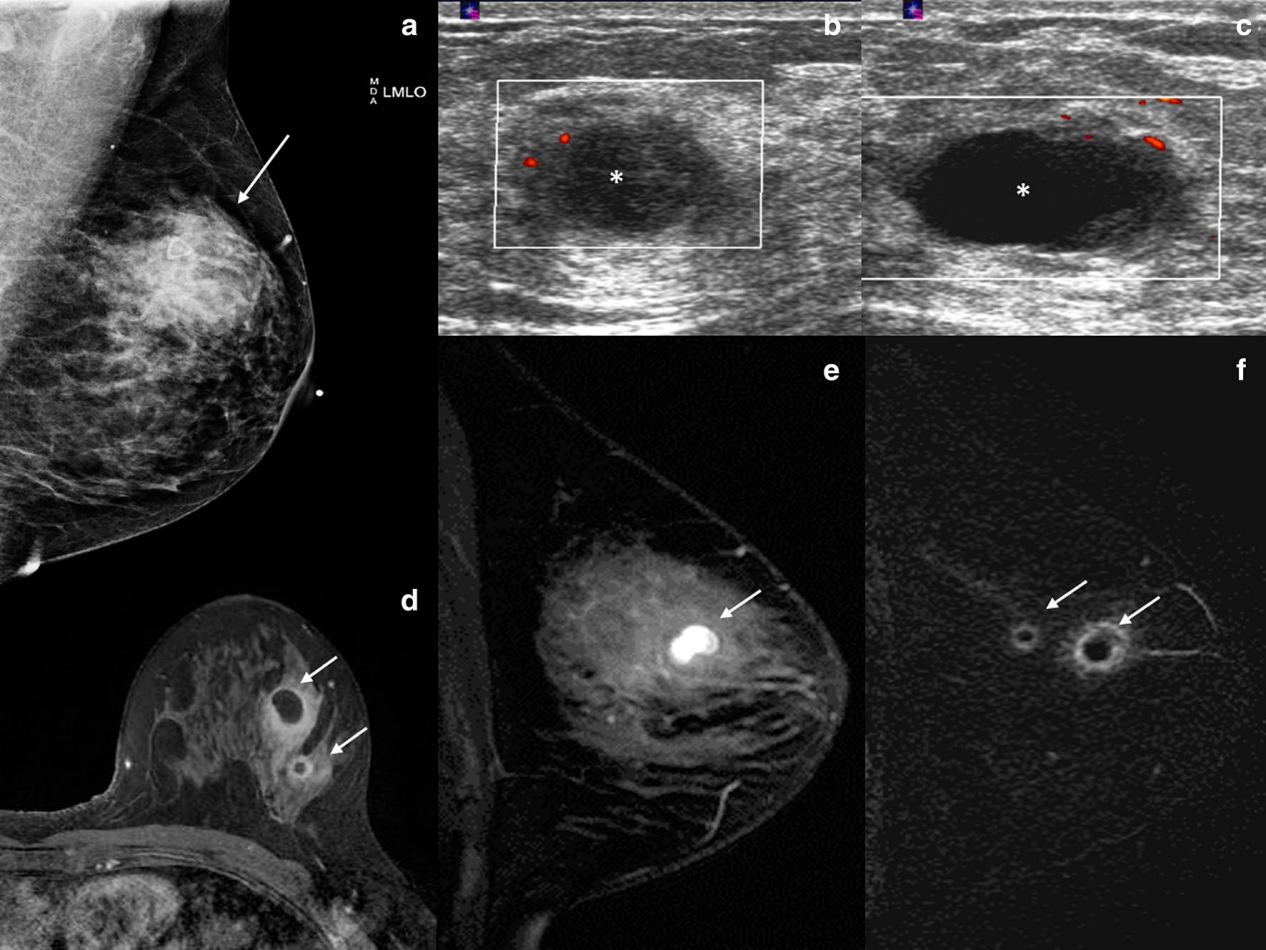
Breast abscess. A 45-year-old woman presented with a palpable area in the right breast. Mediolateral oblique (a) mammogram shows a focal asymmetry in the upper outer breast (arrow) and associated trinagular palpable marker. Power Doppler ultrasound images (b, c) reveal two hypoechoic, oval masses with peripheral vascularity in the same region (asterisks). Axial post-contrast T1-weighted MRI (d), sagittal T2-weighted MRI (e), and postcontrast subtraction T1-weighted MRI (f) show two T2-hyperintense, rim-enhancing masses (arrows). Needle biopsy showed acute inflammatory cells consistent with abscess without evidence of malignancy
